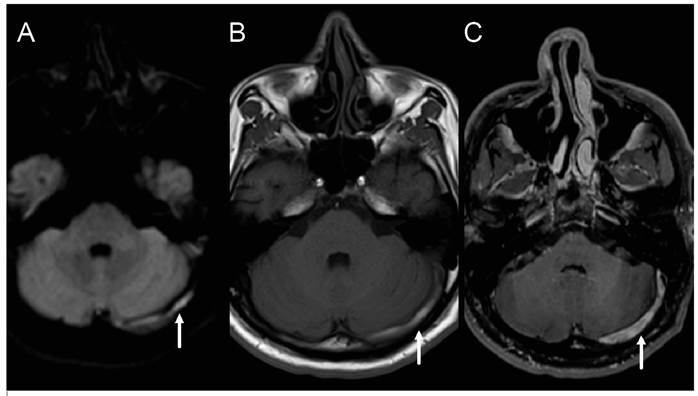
Axial diffusion-weighted images (A), T1-weighted images before contrast administration (B) and post-contrast T1-weighted images (C) showing partial thrombosis of the left transverse sinus (arrows).
